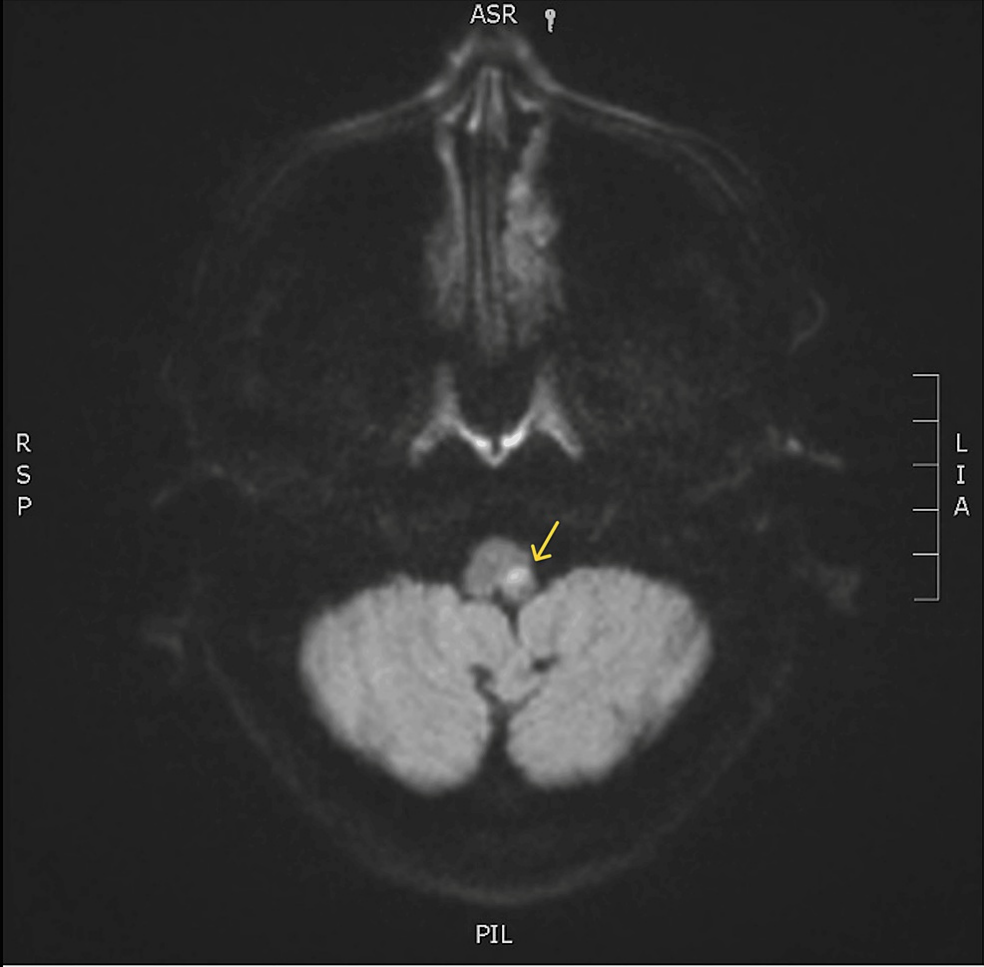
MRI brain image The image reveals a small area of true diffusion restriction with an associated T2 high signal in the left posterolateral medulla, consistent with acute infarct in the left Posterior Inferior Cerebellar Artery (PICA) territory.
